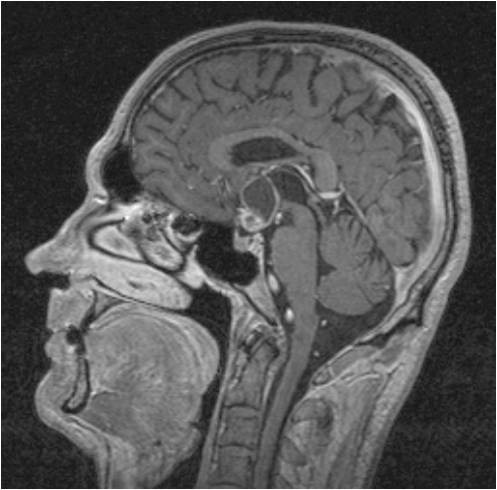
Sagittal brain MRI of a 51 year old male with a recurrence of a craniopharyngioma. The MRI demonstrates the hypertensive solid part and above, it demonstrates the cystic part.
